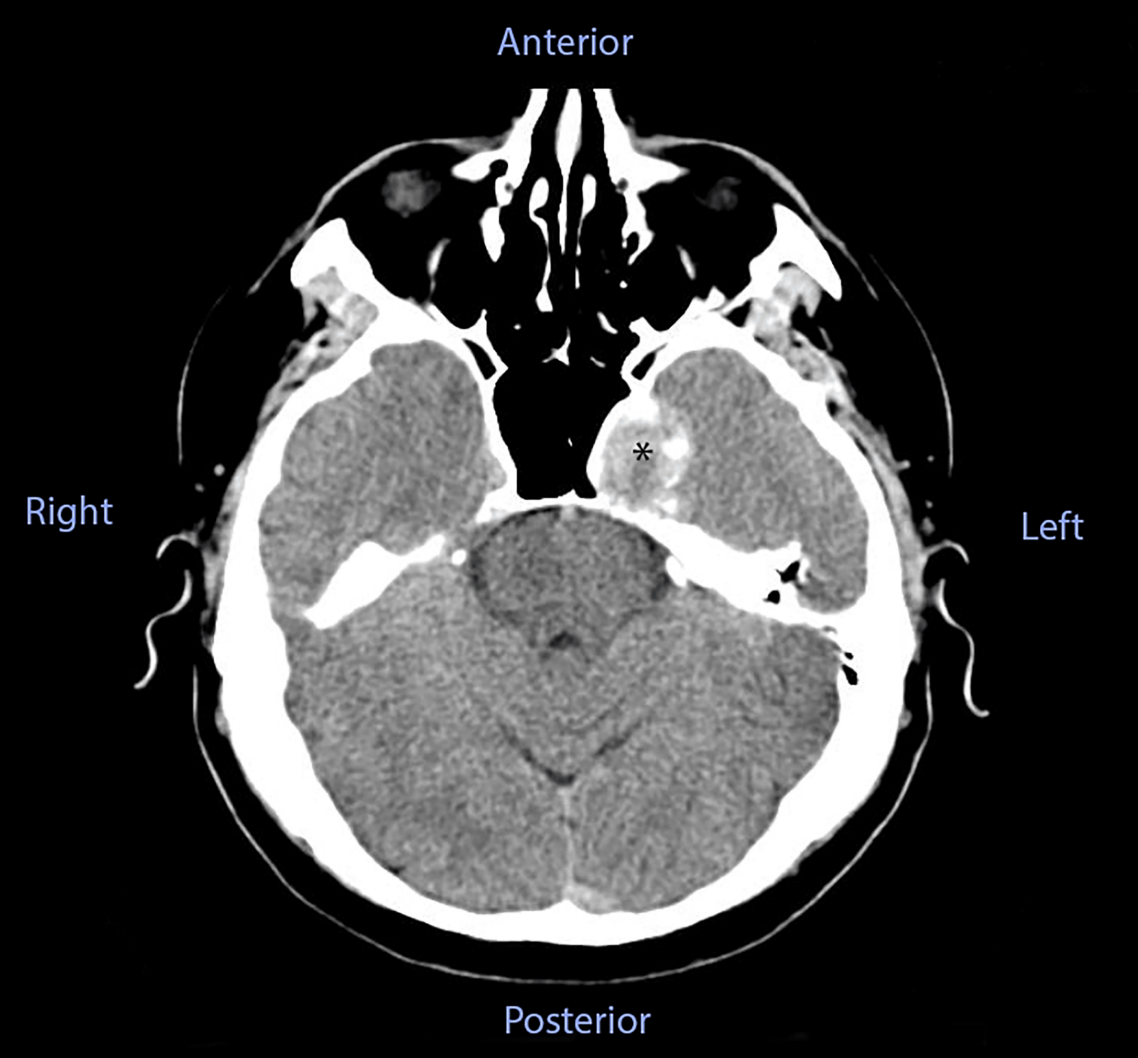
Cerebral CT scan of our patient before being admitted to our hospital. The asterisk marks the discovered lesion on the left side.
